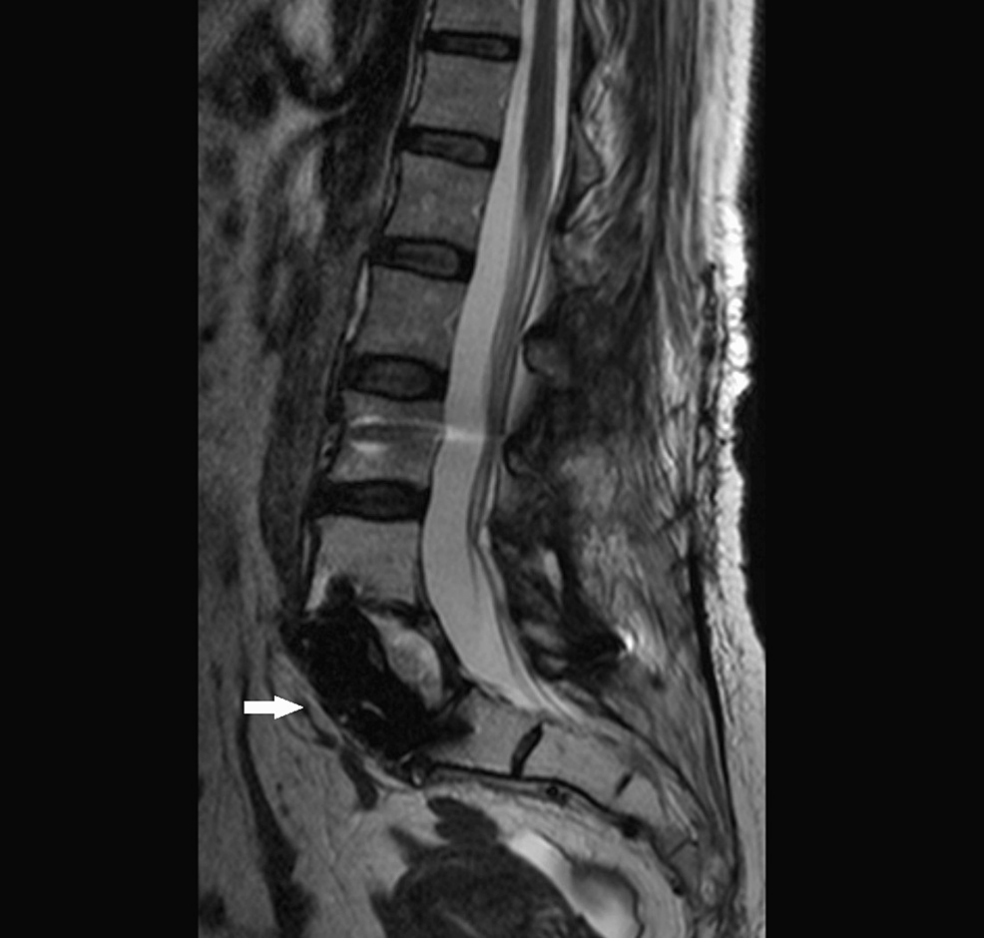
Lumbar MRI after surgery, sagittal T2WI sequence. Lumbar MRI after surgery showed no persistence of disease on sagittal T2WI.
